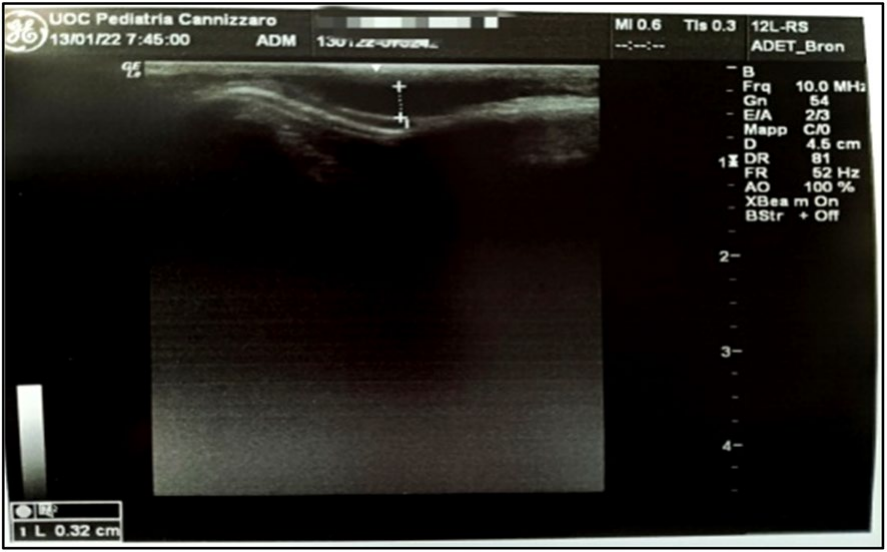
POCUS image, performed at the same time as the CT
